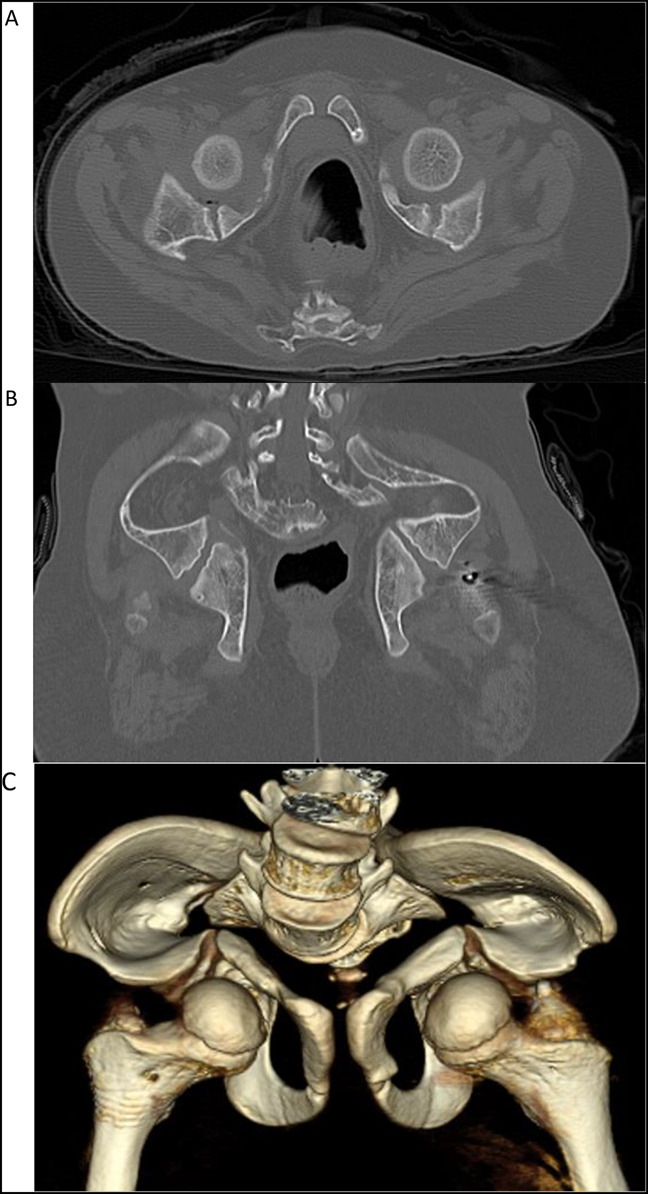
Radiograph showing A, axial and (B) coronal cuts along with (C) 3D reconstruction of pelvis CT obtained at 10 years.
